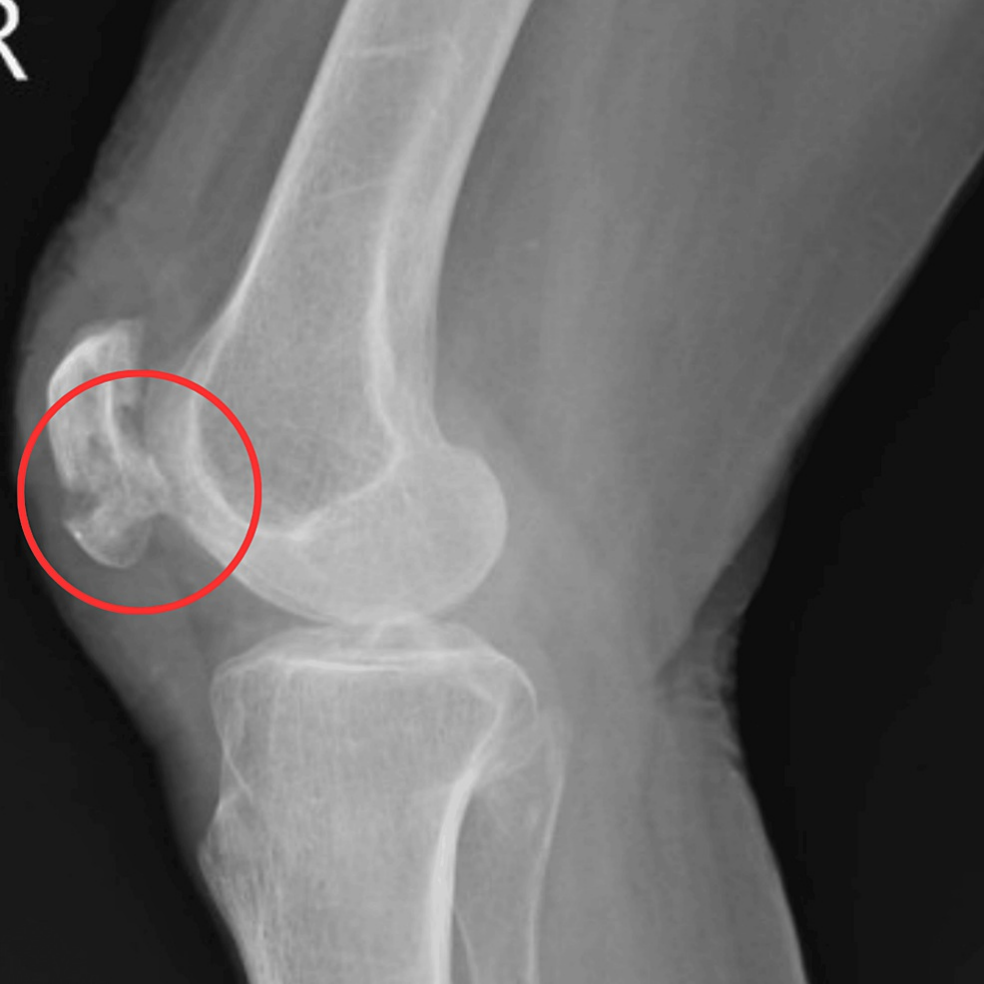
X-ray of the pre-operative patella The red circle shows the fracture of the patella.
